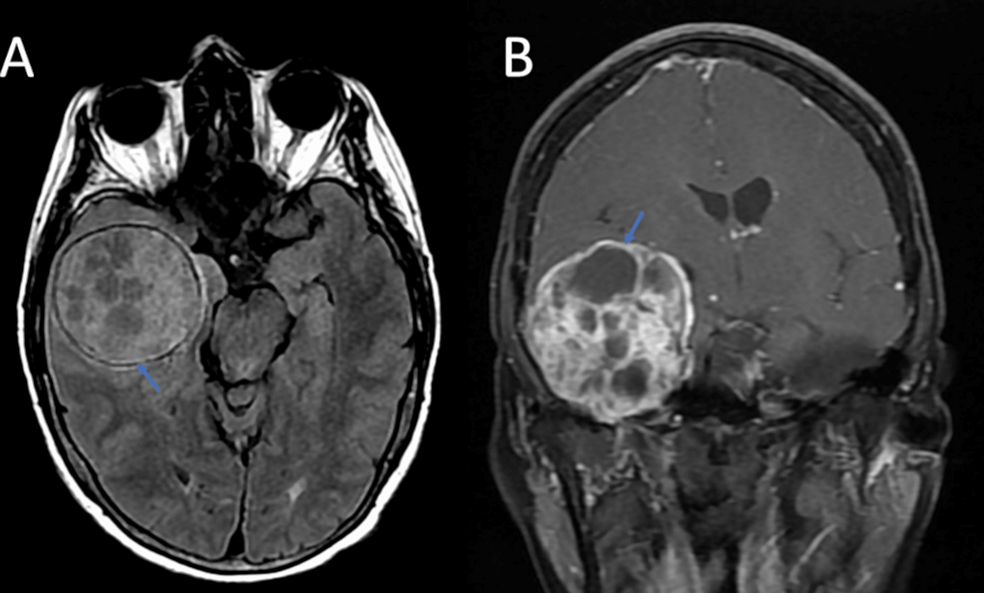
Preoperative MRI: axial T1 without contrast (A) and T1 coronal with contrast (B) Image (A) shows a hypo- to iso-intensity lesion on MRI-T1. Image (B) shows extensive areas of nonenhancement indicating cystic and necrotic changes within the tumor.
